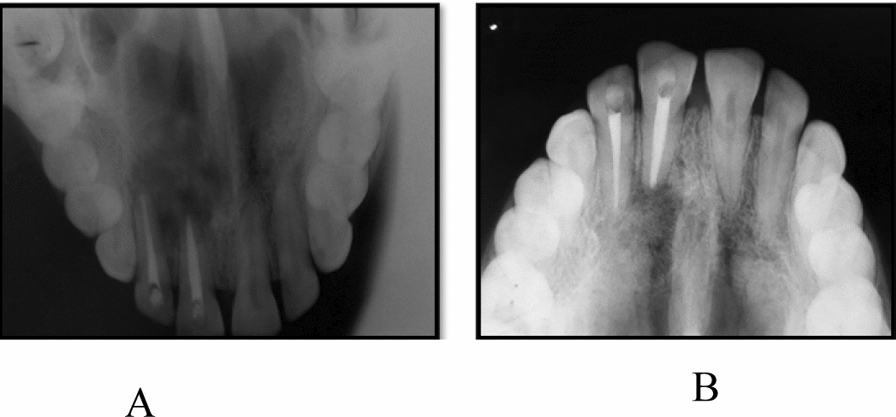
A Radiograph taken after 3 months. B Radiograph taken after 6 months
